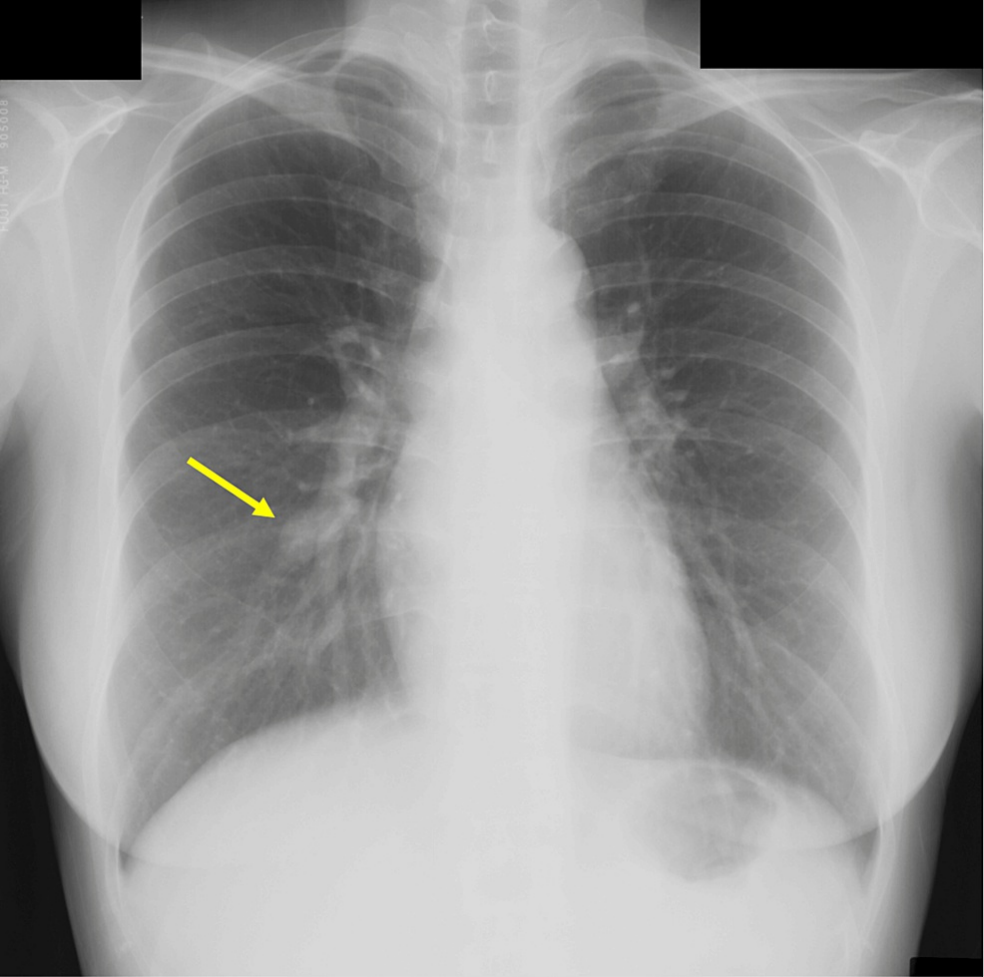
Preoperative imaging findings Chest radiograph shows the nodule in the right lower lung field (yellow arrow).
